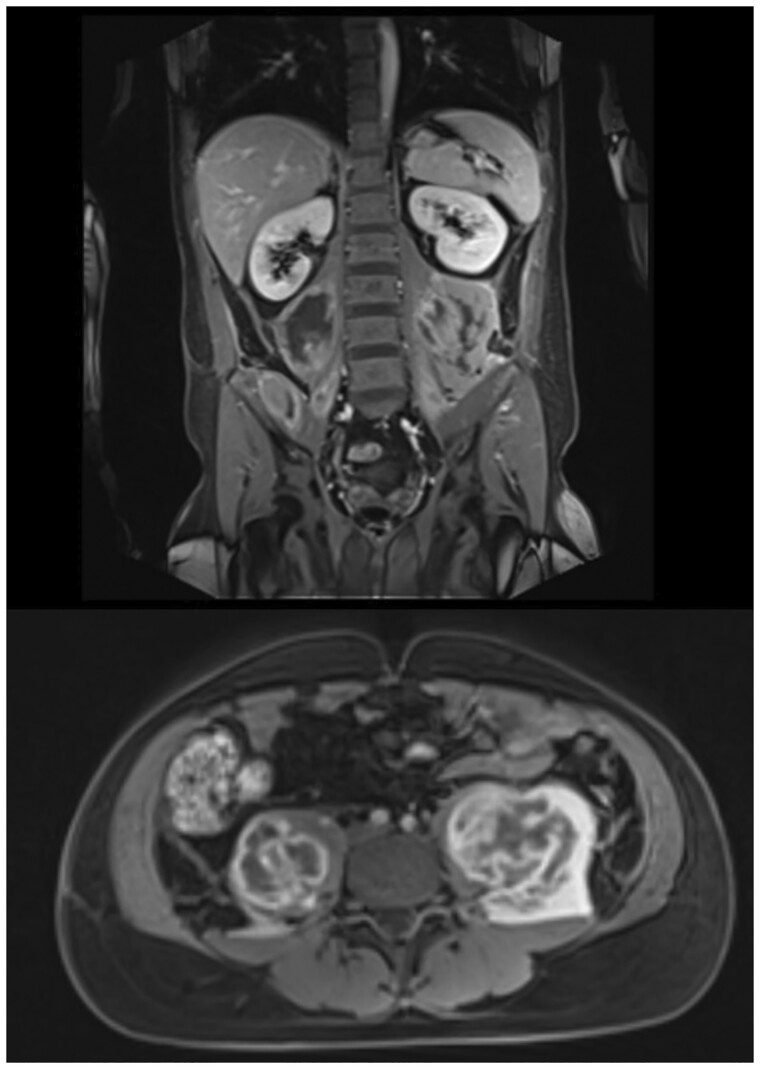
Abdominal MRI after weaning off oxygen showing haemorrhagic infarction of the psoas muscles
